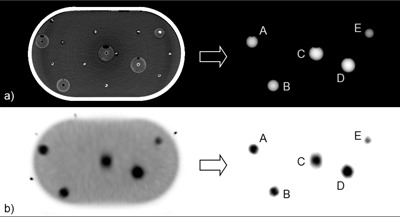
The positions of targets A–E were derived from (a) CT and (b) 12 hr SPECT images and were used subsequently to calculate localization error in the 4.5 min SPECT images. For display purposes, these images have been summed over axial slices containing a target.
